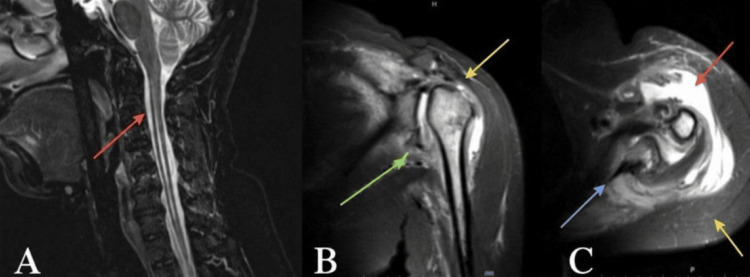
Magnetic resonance imaging findings. (A) Sagittal cervical spine MRI demonstrating an extensive syrinx extending from C2 to C7 in the setting of Chiari I malformation. (B) Coronal oblique shoulder MRI showing humeral head deformity, glenoid erosion, and marked glenohumeral joint effusion. (C) Axial shoulder MRI demonstrating extensive joint and periarticular fluid with rotator cuff muscle atrophy and relative preservation of the deltoid, consistent with neuropathic arthropathy.
